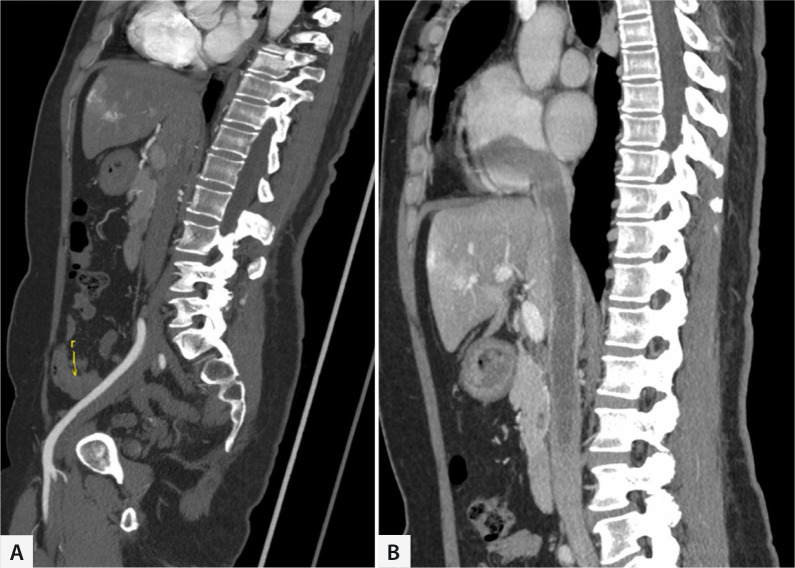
Preoperative computed tomography images of intracardiac leiomyoma. A)
